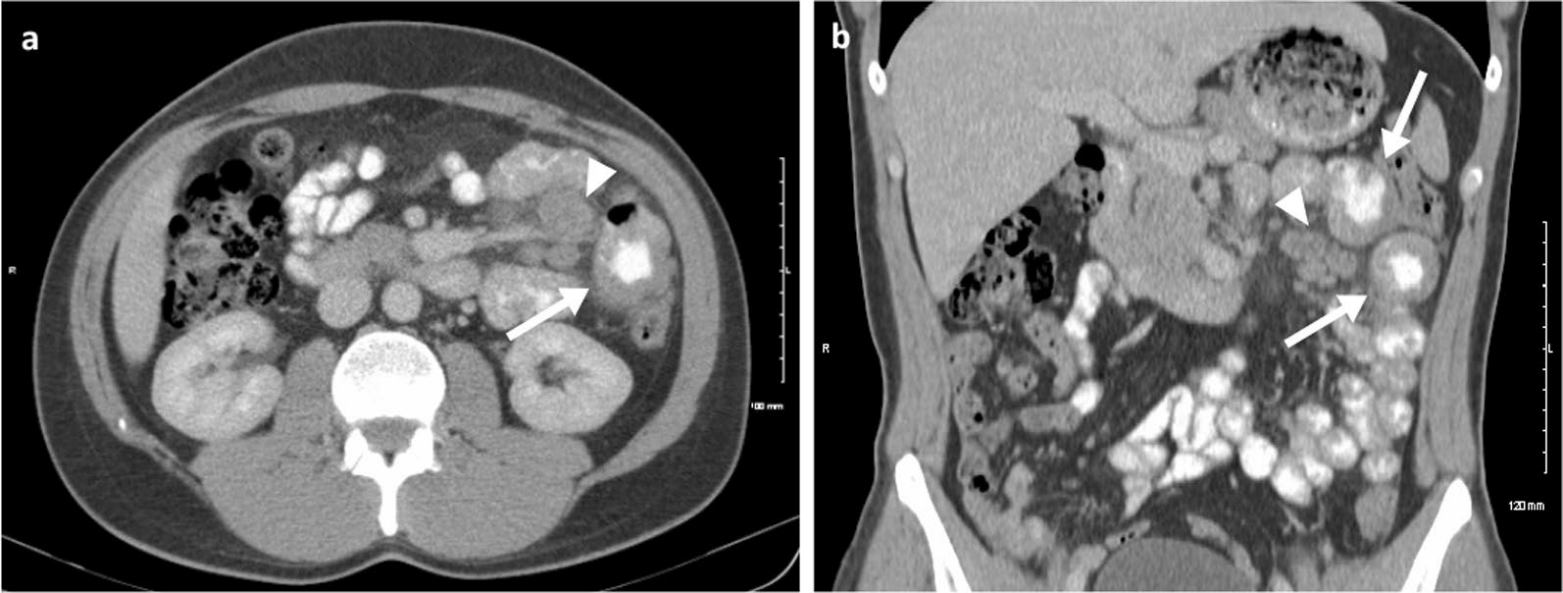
Case report: oral and intravenous portal phase contrast-enhanced CT axial (a) and coronal (b) of a 33-year-old male patient diagnosed with left lower eyelid melanoma which subsequently metastasised to the lymph nodes, lungs, brain, SB and subcutaneous tissue in the following 5-year period. Complete response was achieved after chemotherapy, immunotherapy and radiotherapy. One year after treatment completion he relapsed, presenting with two jejunal metastases (arrows) and involvement of the draining mesenteric lymph nodes (arrow heads). Surgical resection was then performed with no evidence of disease recurrence to date, after 10 years of follow-up
